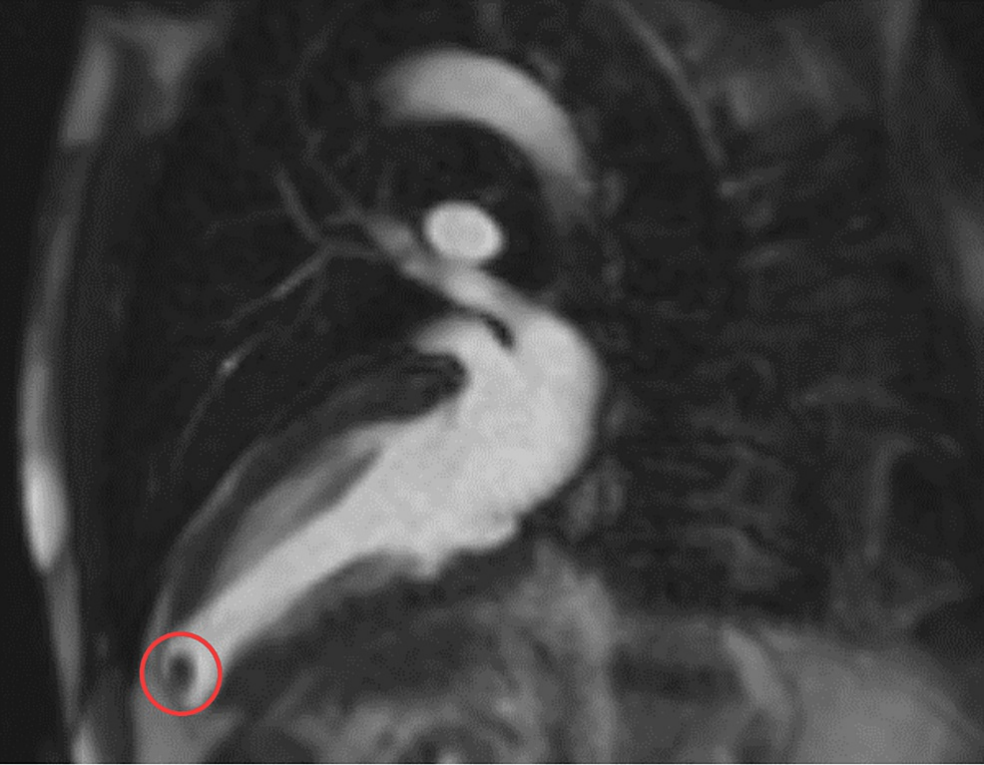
Cardiac MRI during systole. The non-mobile mass is likely a thrombus. MRI, magnetic resonance imaging (poor quality of the image due to some motion artifact)
